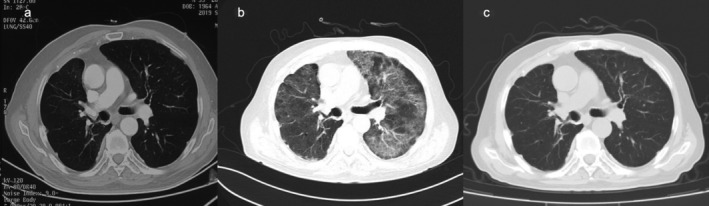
Computed tomography (CT) scan findings in Case 1. (a) Stable disease after two cycles of nivolumab and docetaxel. (b) Diffuse ground‐glass opacities (GGOs) were visible bilaterally. (c) Radiographic lesions resolved markedly after antimicrobial and glucocorticoid treatment.
